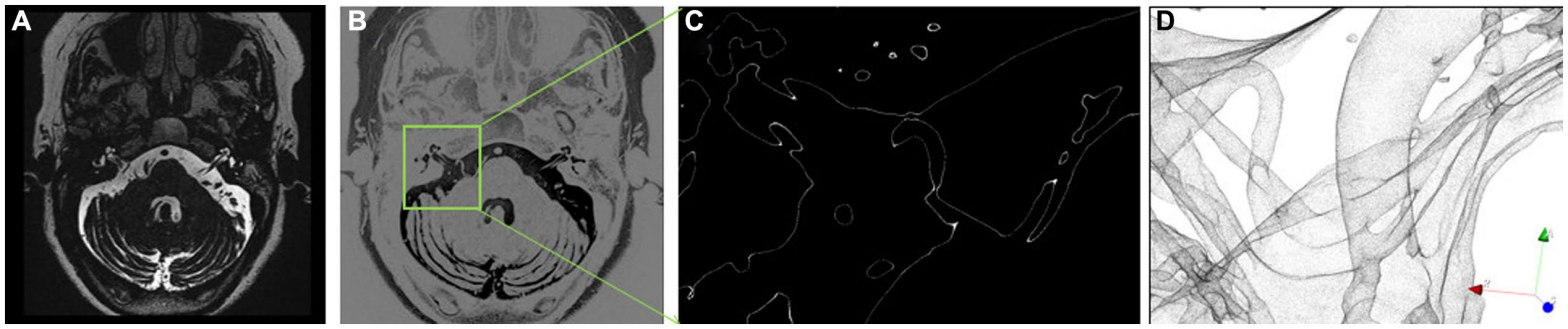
Non-segmented inverted water outline technique. (A) MR image, (B) inverted grayscale image; (C) extraction of water outline within ROI; (D) three-dimensional rendering based on water outline.
